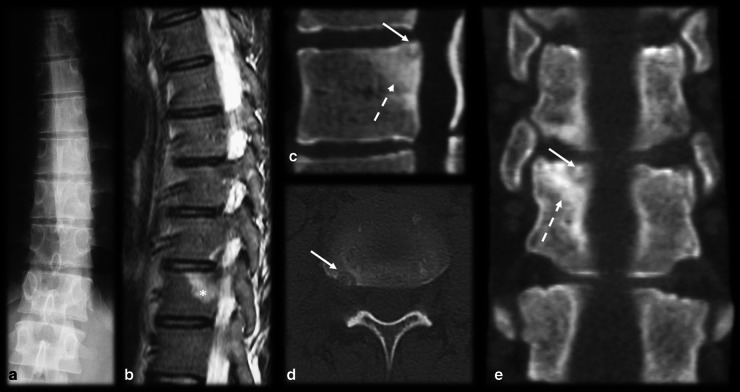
Osteoid osteoma mimicking enthesitis. Male, 20 years old, presenting with back pain for six weeks. CR (a) showed left scoliosis with no identifiable bone lesions. Sagittal T2 FS MRI (b) revealed bone marrow edema on the posterosuperior corner of the vertebral body (asterisk), which raised the suspicion for enthesitis. The CT scan (c, d, e) showed a small and mineralized nidus on the concave side of the region of scoliosis (arrow) with reactional bone sclerosis (dashed arrow)
